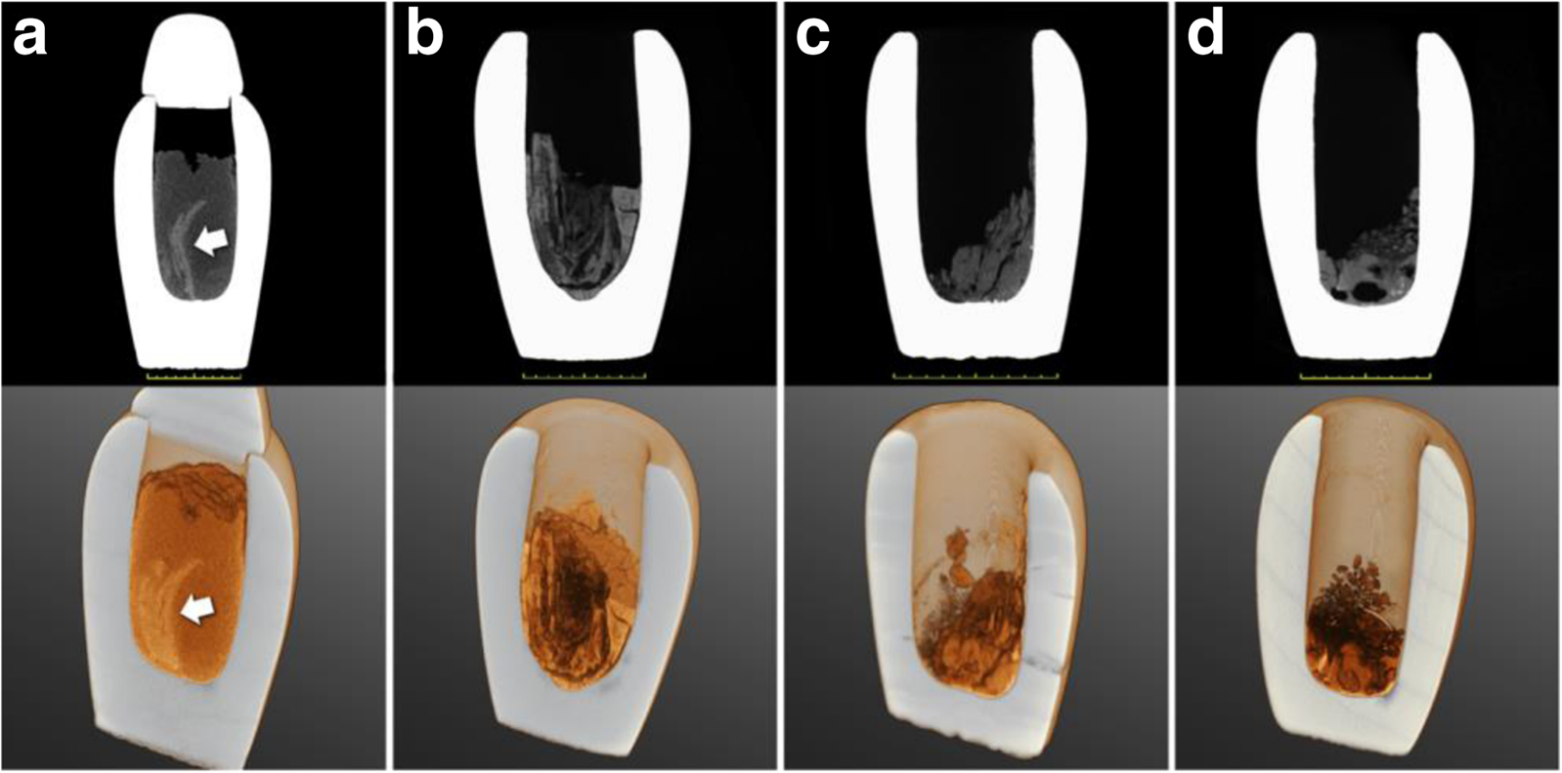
CT scans, sagittal cross sections through the centre of the jars (*top*) and correlative 3D volume renderings (*bottom*) of all four canopic jars (a, inventory number 607; b, inventory number 617; c, inventory number 622-1; d, inventory number 610-2). In panel a, *arrows* indicate a distinct hyperdense structure of organ-fragment-like morphology, probably intestine
